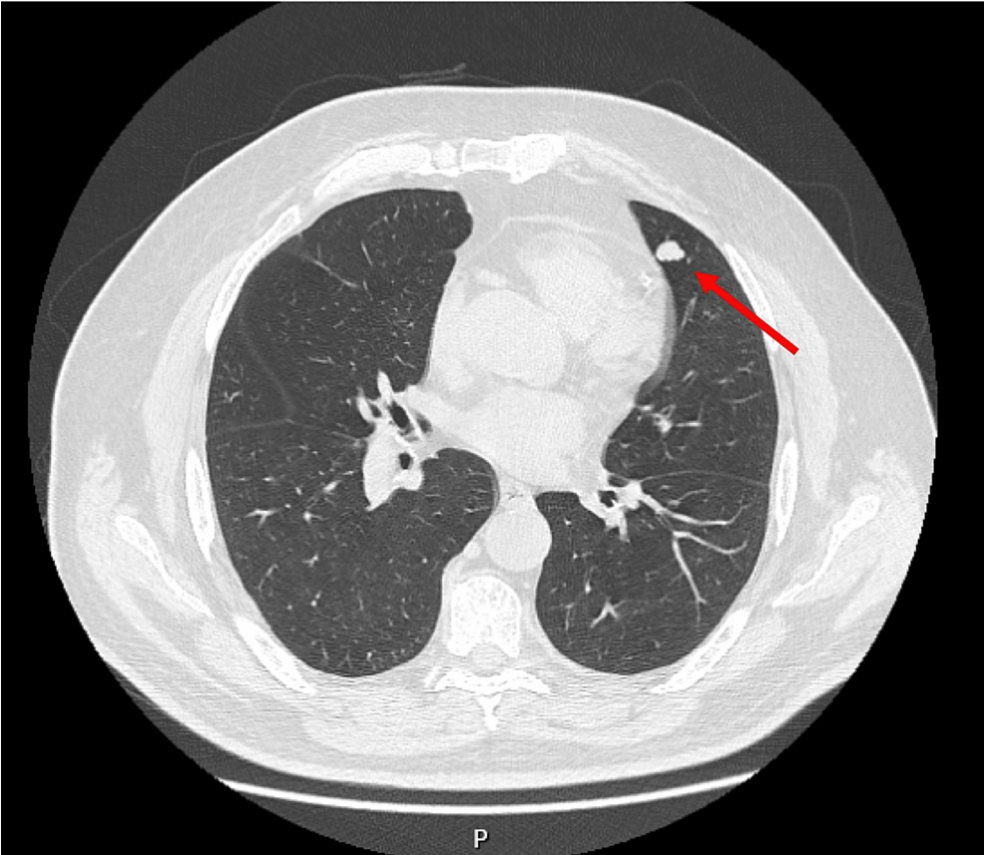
Chest CT with a pulmonary nodule detected.
